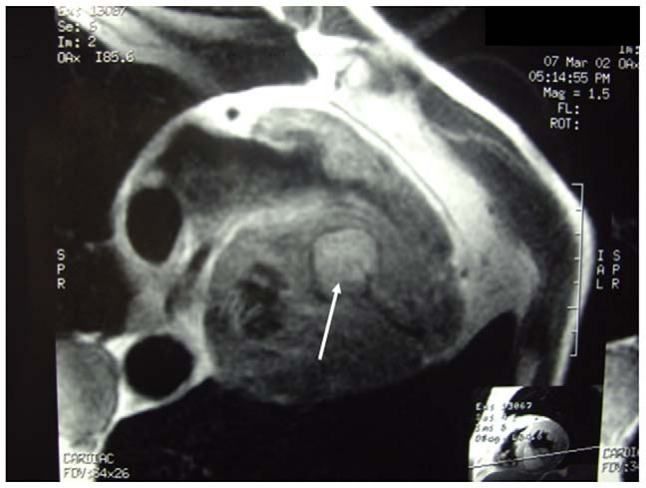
Computed tomography showing CE in intramyocardial interventricular septum (arrow) (patient 1).
